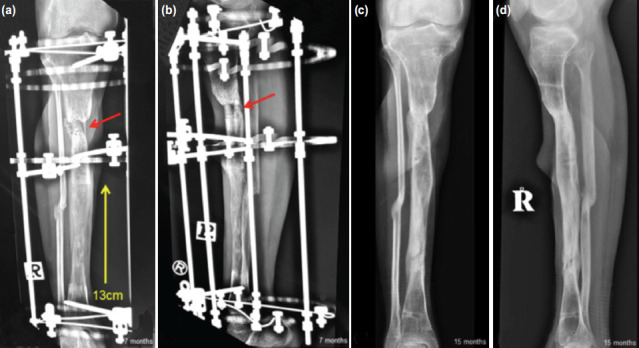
(a) Anteroposterior and (b) lateral radiographs of the right tibia at seven months following bone transport with the Ilizarov method. Red arrows showing multiple drilling over the docking site performed two months previously with injections of peripheral blood stem cells to enhance callus formation. Yellow arrow indicating the direction of bone transport from the ankle corticotomy site, showing 13cm of bone regeneration over the distal half of the tibia. Similar views (c) and (d) at 15 months, showing bone healing at the docking site and further consolidation of the regenerated bone.
